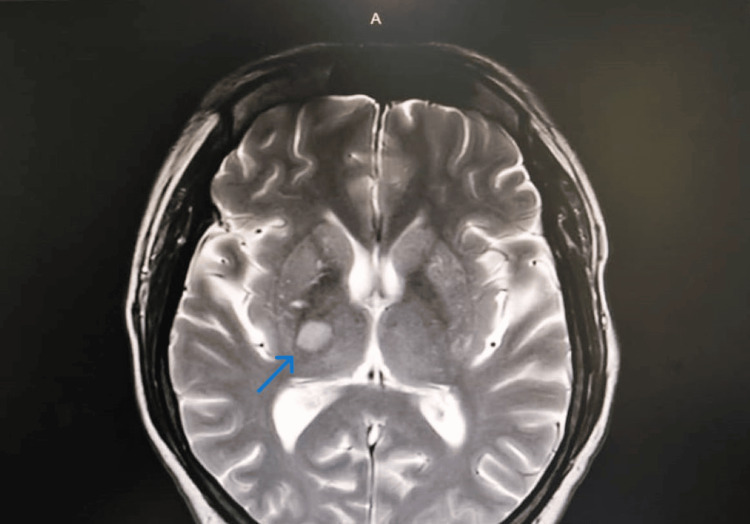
MRI shows restricted diffusion to right thalamus and posterior internal capsule (arrow).
